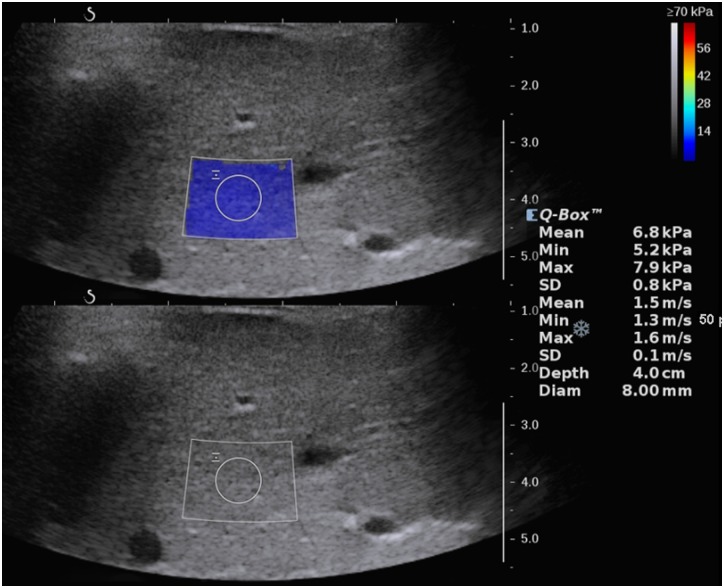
Ultrasound elastography image obtained from the liver of a 6-year-old girl. The circular region of interest was placed on the color-coded map of homogeneous liver parenchyma at a location that avoided vessels and bile ducts in the right lobe. The child was in the supine position with free breathing status. Mean values were simultaneously provided for both units of elasticity and velocity as 6.8 kPa and 1.5 m/sec.
